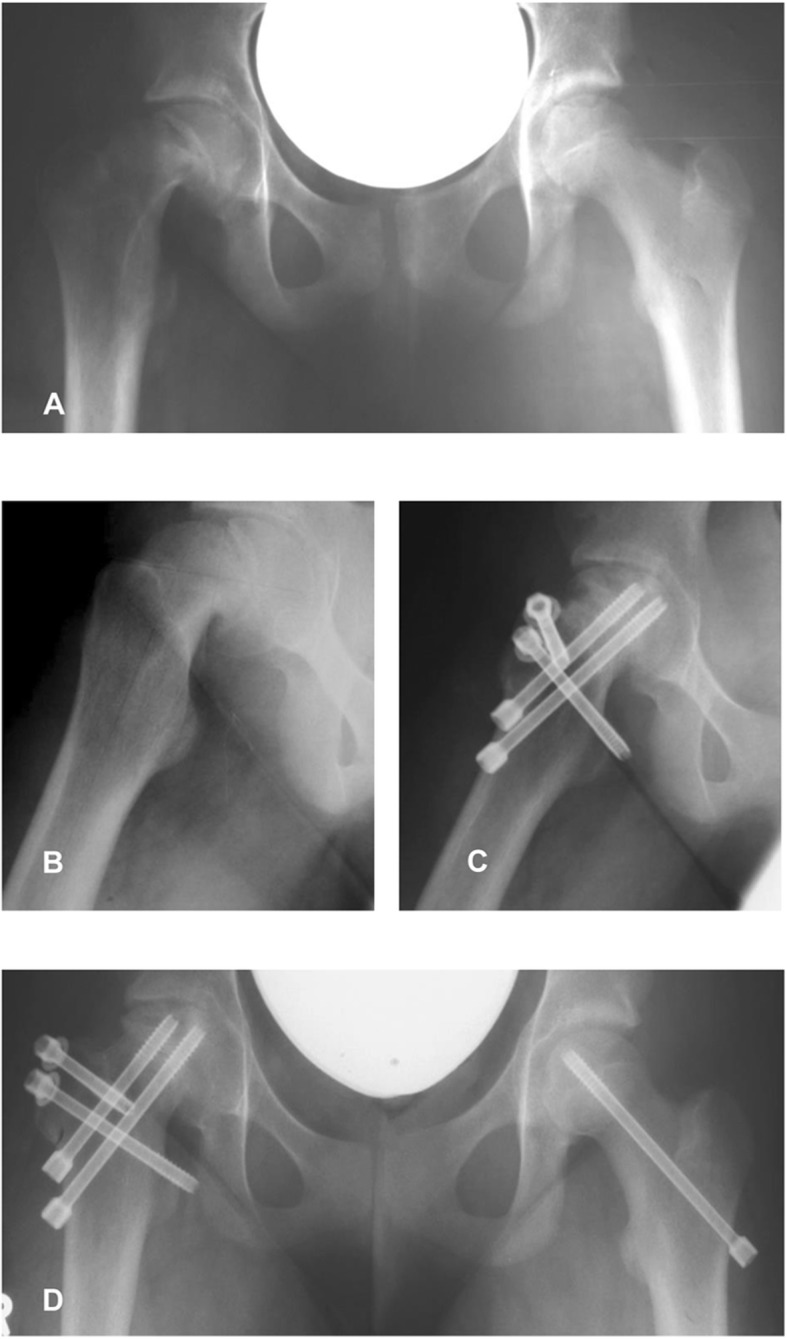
Preoperative (a, b) and 1 year postoperative (c, d) X-rays of a 12-year-old female patient with an acute on chronic SCFE with an SSA of 78°. This patient developed a partial avascular necrosis of the femoral head
